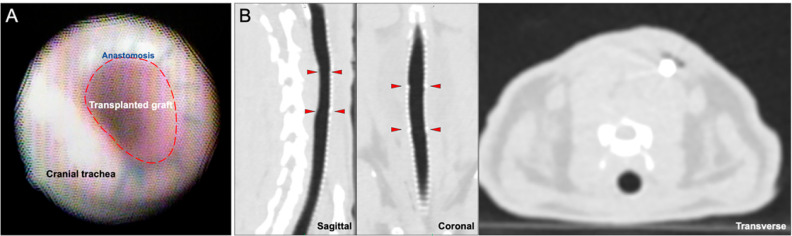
Bronchoscopic and Radiographic Surveillance at 7 Days Post-transplant. (A) Bronchoscopy shows a patent tracheal graft without evidence of necrosis. (B) Sagittal, coronal, and transverse views show intact tracheal anastomosis without stenosis or extraluminal air suggestive of dehiscence. The red arrowheads indicate the proximal and distal anastomotic boundaries.
